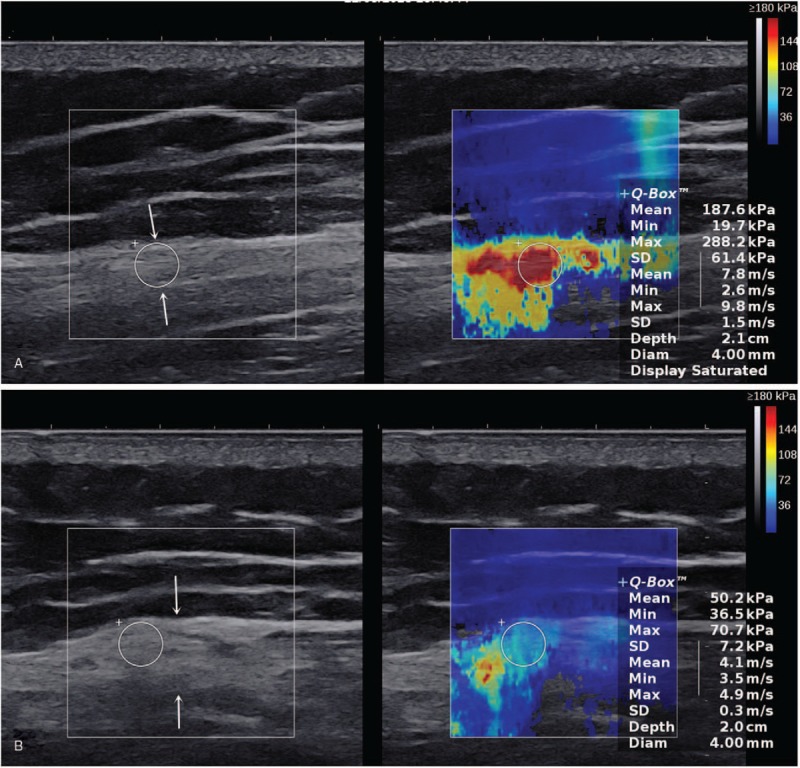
A 28-year-old woman with gluteal muscle contracture. This figure shows the gray-scale ultrasound image and shear-wave elastogram of the contracture strip (arrows). (A) Longitudinal view of the gluteus maximus muscle. (B) Transverse view of the gluteus maximus muscle.
